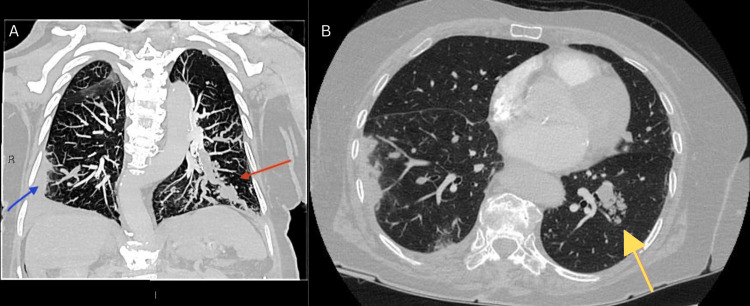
CT pulmonary angiogram completed three months after the initial presentation: (A) maximum intensity projection coronal view and (B) axial view. Coronal CT image demonstrates an occlusive tumour in the lateral basal segmental pulmonary artery with an irregular beading appearance, branching centrilobular nodules, and persisting luminal distension (red arrow). A peripheral lung infarct can also be seen in the right lower lobe (blue arrow). Axial CT image demonstrates persisting tree-in-bud pattern evident in in the lateral basal segment of the left lower lobe (yellow arrow).
